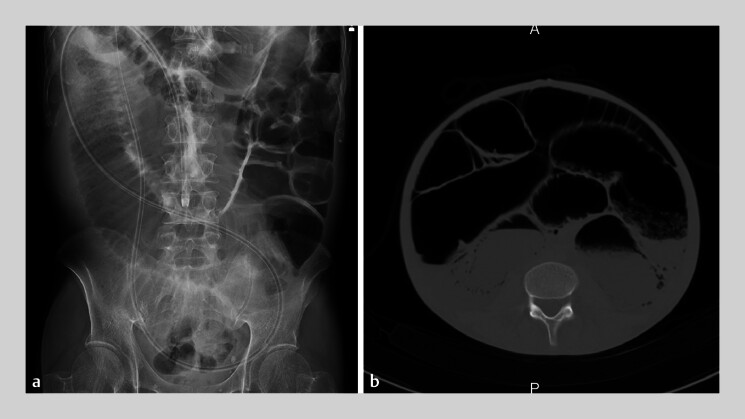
Pre-admission abdominal radiography and computed tomography images.
